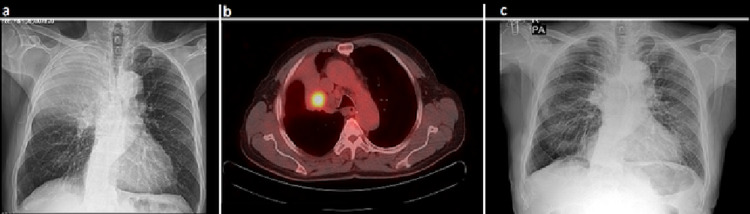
A chest x-ray showing the right upper lobe atelectasis (a), PET-CT showing a pathologically increased uptake of 18F-FDG on the mass (b), and the non-complicated chest x-ray taken at the discharge of the patient (c).
